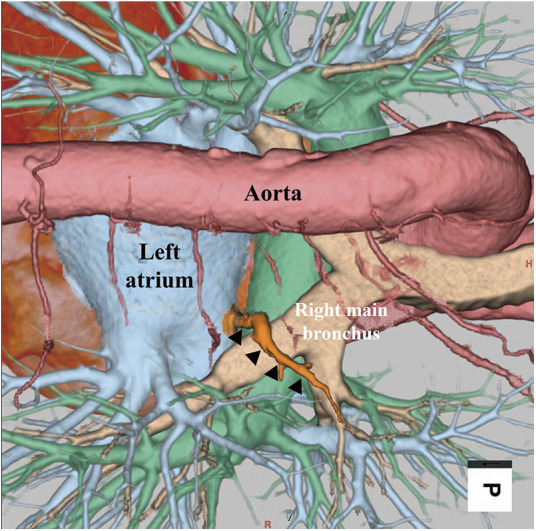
3D-CT imaging. The anomalous RSPPV (arrowheads) runs behind the right intermediate bronchus and flows into RSPV. RSPPV: right superior posterior pulmonary vein
